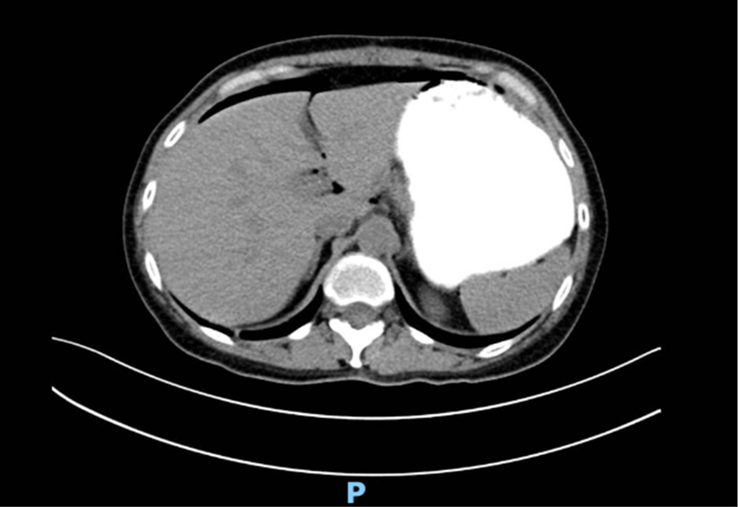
Axial computed tomography scan of the abdomen showing free peritoneal air.
